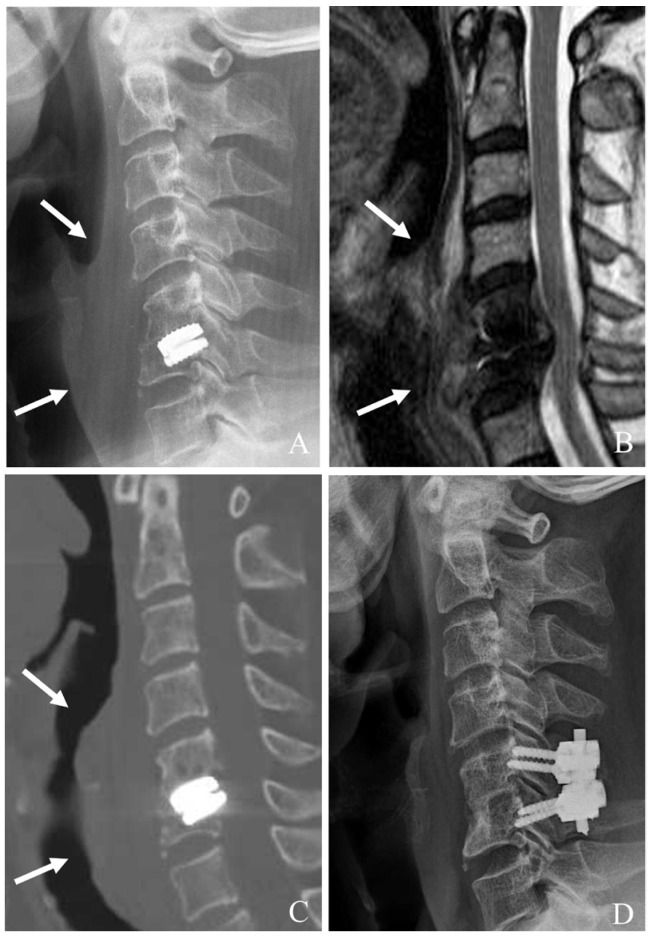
Plain X-rays (A), magnetic resonance image (B), and computed tomography (C) showing subsidence and retropulsion of cervical disc arthroplasty at C5–6 with retropharyngeal (white arrows) and epidural abscess causing cord compression. The patient underwent the removal of the C5–6 prosthesis and combined C5–6 anterior cervical discectomy and fusion and posterior fusion with lateral mass screws (D).
